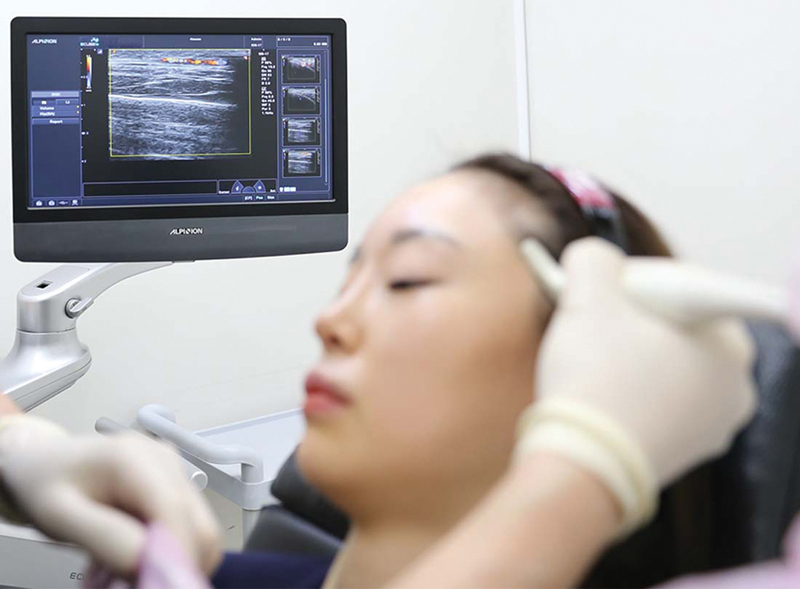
Doppler ultrasound detection of the frontal branch of superficial temporal artery at temple area.
